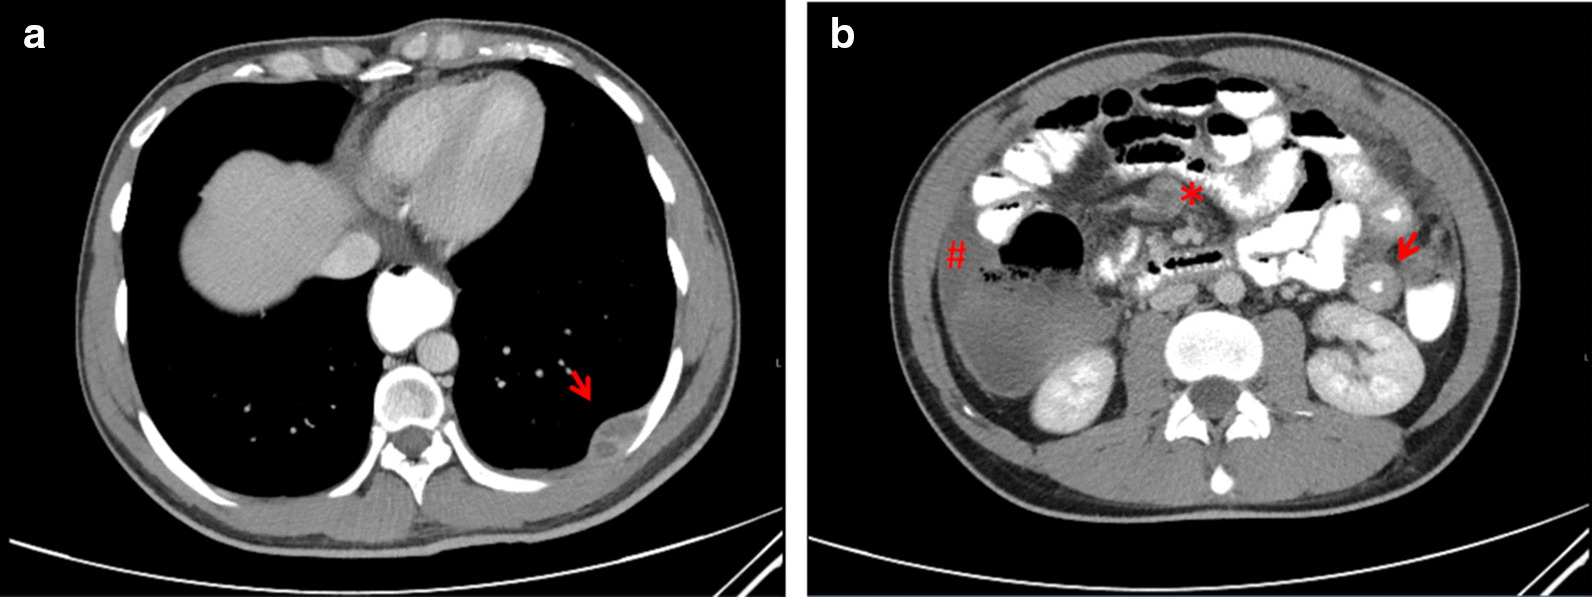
Initial computed tomography scan demonstrating (a) a pleural mass (the distal esophagus is distended as a secondary finding) and (b) necrotic abdominal lymph nodes (*), bowel wall thickening (→), and ascites (#)
